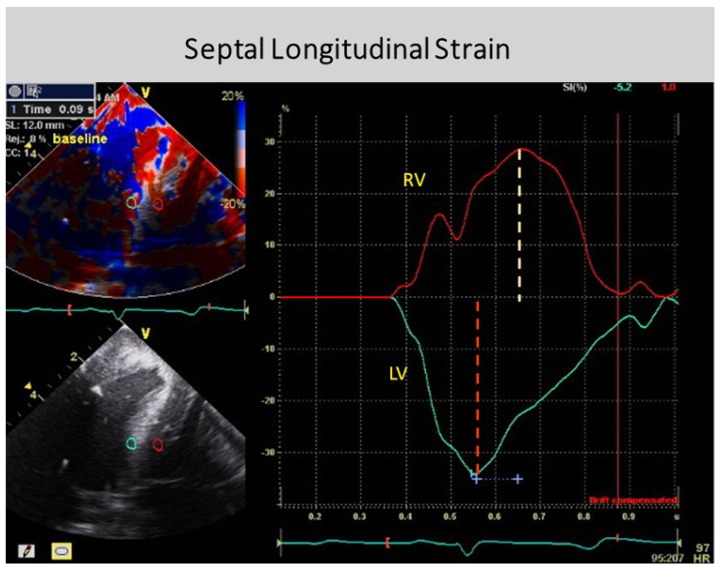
Doppler longitudinal strain imaging of septum (right and left sides) in apical four-chamber view. Longitudinal strain marked by red (right) and green (left) circles, showing deformation in opposite directions on right and left septum sides—relative to baseline zero value. Timing shows LV first and RV second. SR, Strain rate; AVC, aortic valve closure; RV, right ventricle; LV, left ventricle. Reproduced form the reference [38] with Publisher’s permission.
